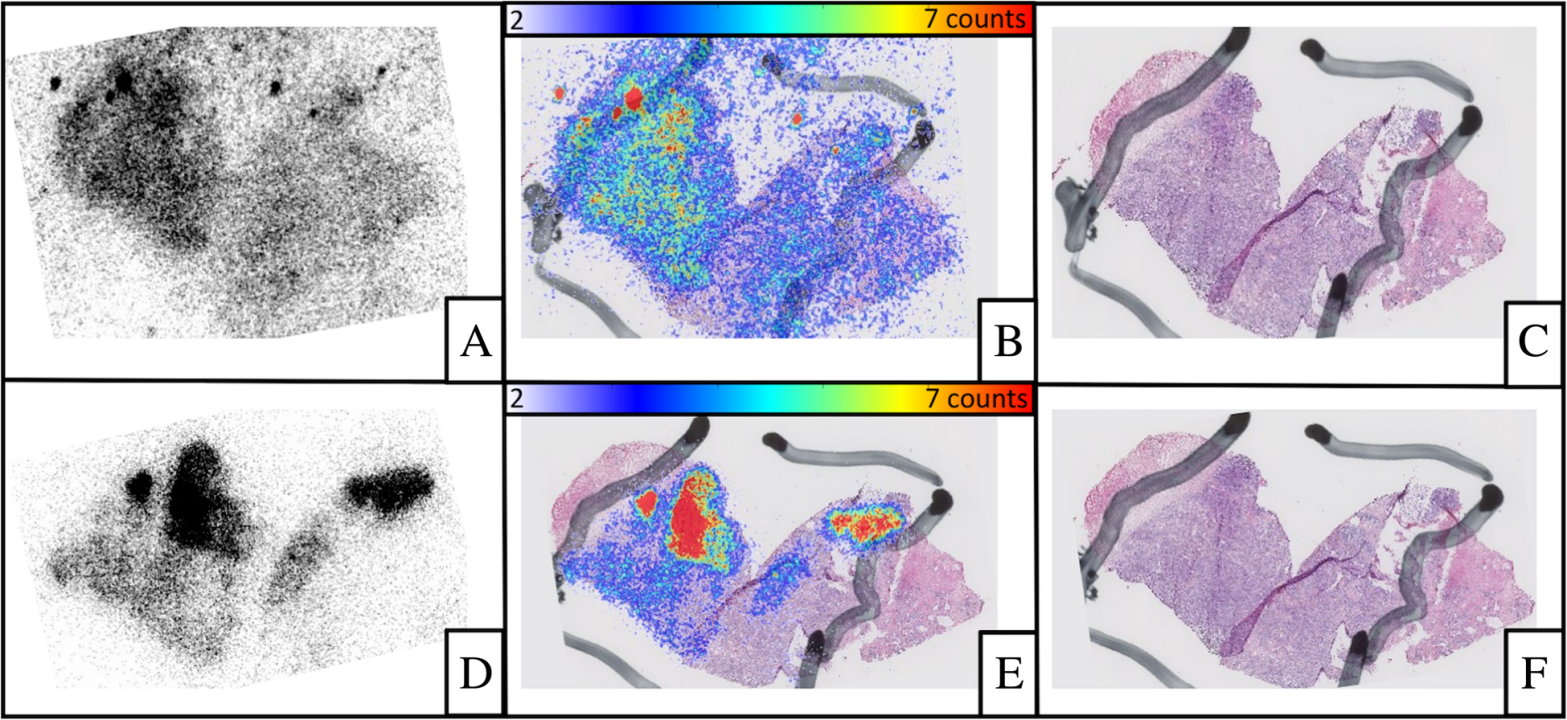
Comparison between ^111^In-RM2 (a–c) and ^111^In-PSMA (d–f) on a high-risk sample: radioactive signal (a, d), HES (c, f), and fusion images (b, e). The black line delimitation corresponds to the tumoral area. There is excellent discrimination between tumor tissue and normal tissue on ^111^In-PSMA-617 (TNR = 11.20), while the contrast is somewhat lower with ^111^In-RM2 (TNR = 1.21)
